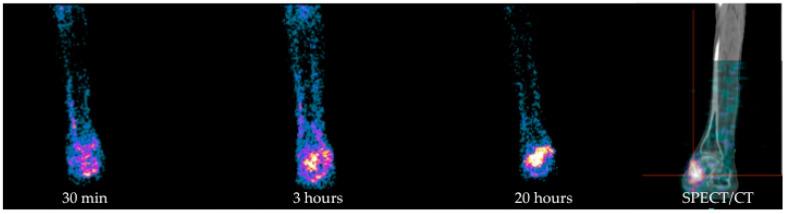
Example of ^99m^Tc-HMPAO WBC scintigraphy in a patient with a skin ulcer in the medial right malleolus region, previous amputations of left leg, and of all metatarsal heads of the right foot. From left to right: Planar images acquired after 30 min, 3 h, and 20 h p.i. of radiolabelled autologous leukocytes show an increased amount of activity over time in terms of intensity and extent, being consistent with OM with an involvement of adjacent soft tissues. Further SPECT/CT acquisition (right panel) correctly localized the uptake in the right talus and accurately evaluated its extent into surrounding soft tissues. HMPAO: hexamethylpropylene amine oxine, WBC: white blood cell.
